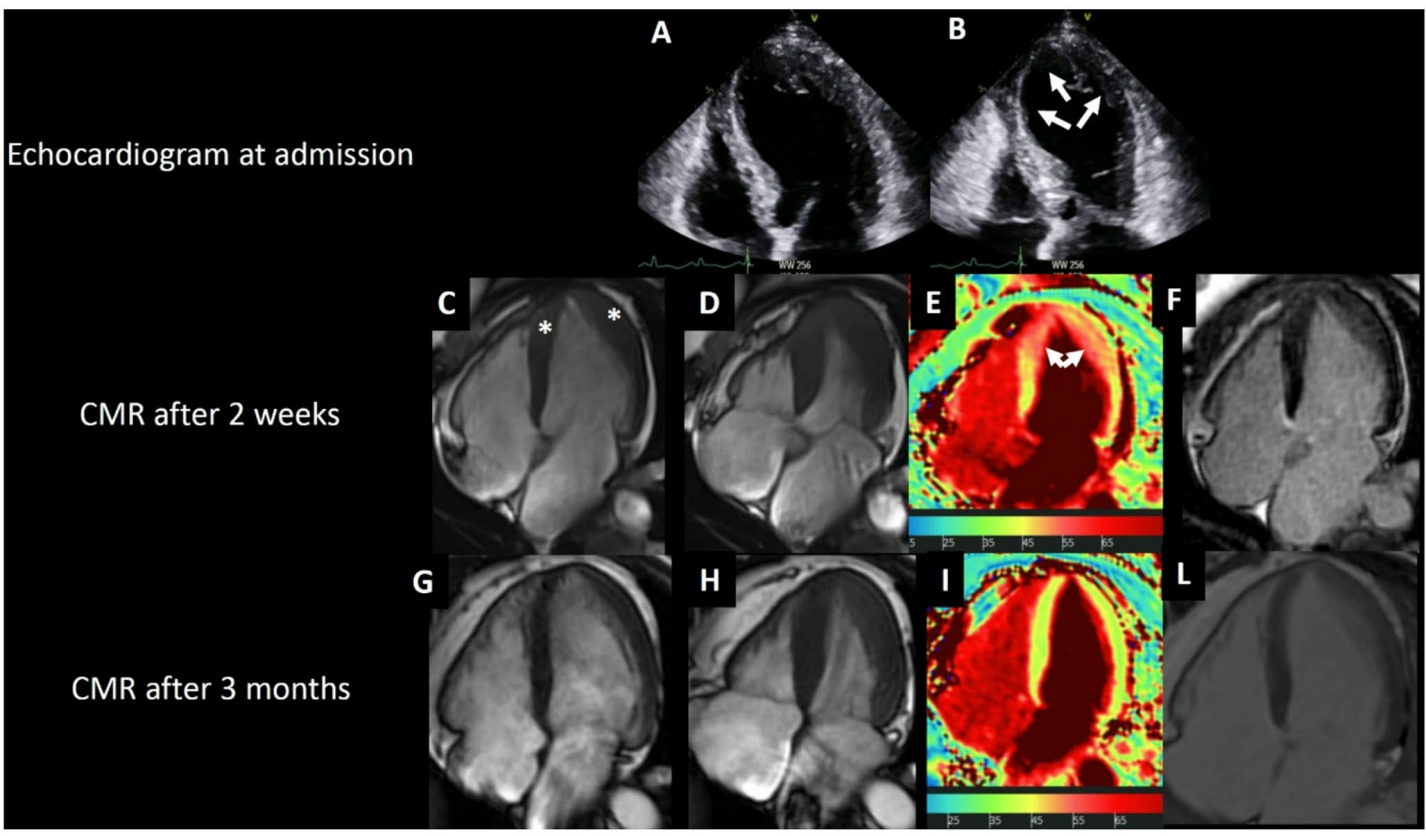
Temporal evolution of pseudo-apical hypertrophic cardiomyopathy phenotype in Takotsubo cardiomyopathy. Panels A-B: Echocardiogram performed at admission. Four-chamber end-diastolic (A) and end-systolic (B) frames showing mid-apical left ventricular akinesia with apical ballooning. Panels C-F: Cardiac magnetic resonance imaging performed two weeks after presentation. Four-chamber cine CMR images in end-diastole (C) and end-systole (D) show normalised ejection fraction with increased apical wall thickness (*; 13 mm) creating a pseudo-apical hypertrophic cardiomyopathy appearance. T2 mapping (E) demonstrates elevated T2 values (arrows; up to 60 ms) in the apical segments, indicating myocardial oedema and inflammation. Four-chamber late gadolinium enhancement imaging (F) shows no myocardial scar or replacement fibrosis. Panels G-L: Follow-up cardiac magnetic resonance imaging at 3 months. Four-chamber cine CMR images in end-diastole (G) and end-systole (H) show complete normalisation of myocardial thickness with preserved normal ejection fraction. T2 mapping (I) indicates normalised T2 values, signifying resolution of myocardial oedema. Late gadolinium enhancement imaging (L) continues to reveal absence of myocardial scar
